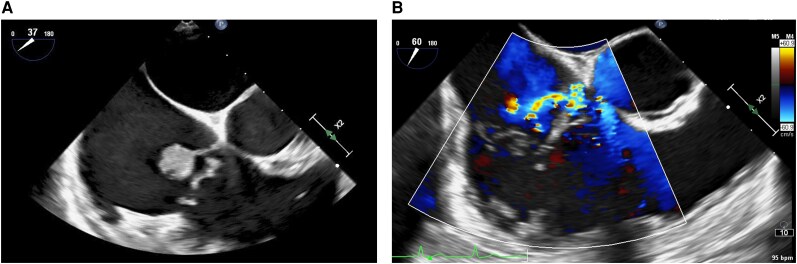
Transoesophageal echocardiography demonstrated a highly mobile, club-shaped vegetation (18.6 × 10 mm) adjacent to the tricuspid valve (*A*). Severe tricuspid regurgitation due to septal leaflet rupture was observed (*B*). Transoesophageal echocardiography also confirmed no patent foramen ovale on colour Doppler imaging and no left heart-sided vegetation.
